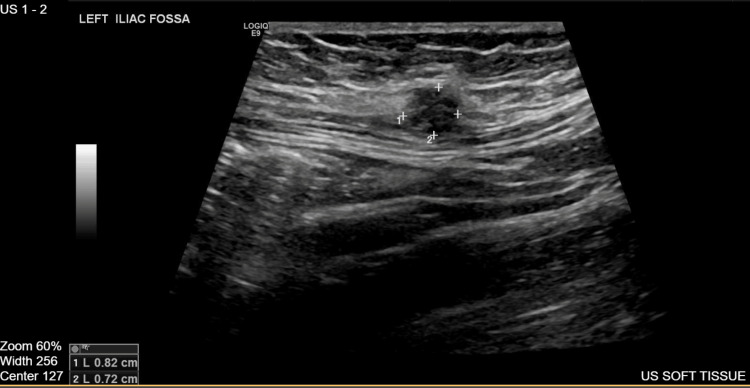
Soft tissue ultrasound showing a cystic lesion in the left rectus muscle.
